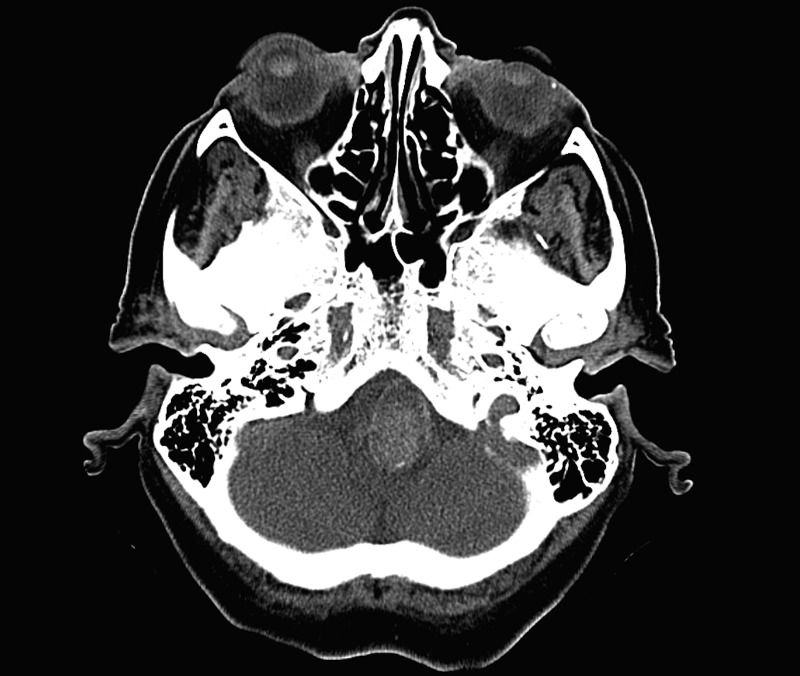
February Axial CT Image 2 Posterior fossa
